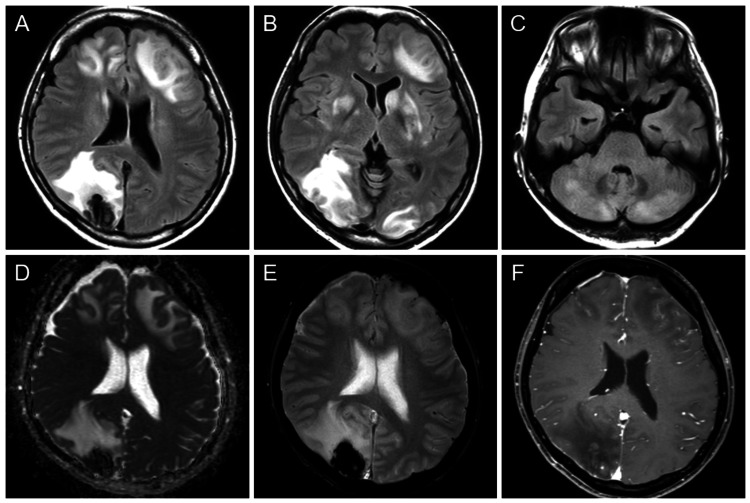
Brain magnetic resonance imaging performed after the seizure on the ninth day of hospitalization. (A-C) Fluid attenuated inversion recovery images show hyperintensity and swelling in multiple areas, including the cortex and deep white matter of the frontal, parietal, and occipital lobes, as well as the basal ganglia and cerebellum. (D-F) The lower-row figures represent apparent diffusion coefficient (ADC) map (D), T2*-weighted image (E), and contrast-enhanced fat-suppressed T1-weighted image (F), all corresponding to the same slice level as shown in Panel A. The lesions are accompanied by hemorrhage and meningeal enhancement. The non-hemorrhagic areas show increased ADC values.
